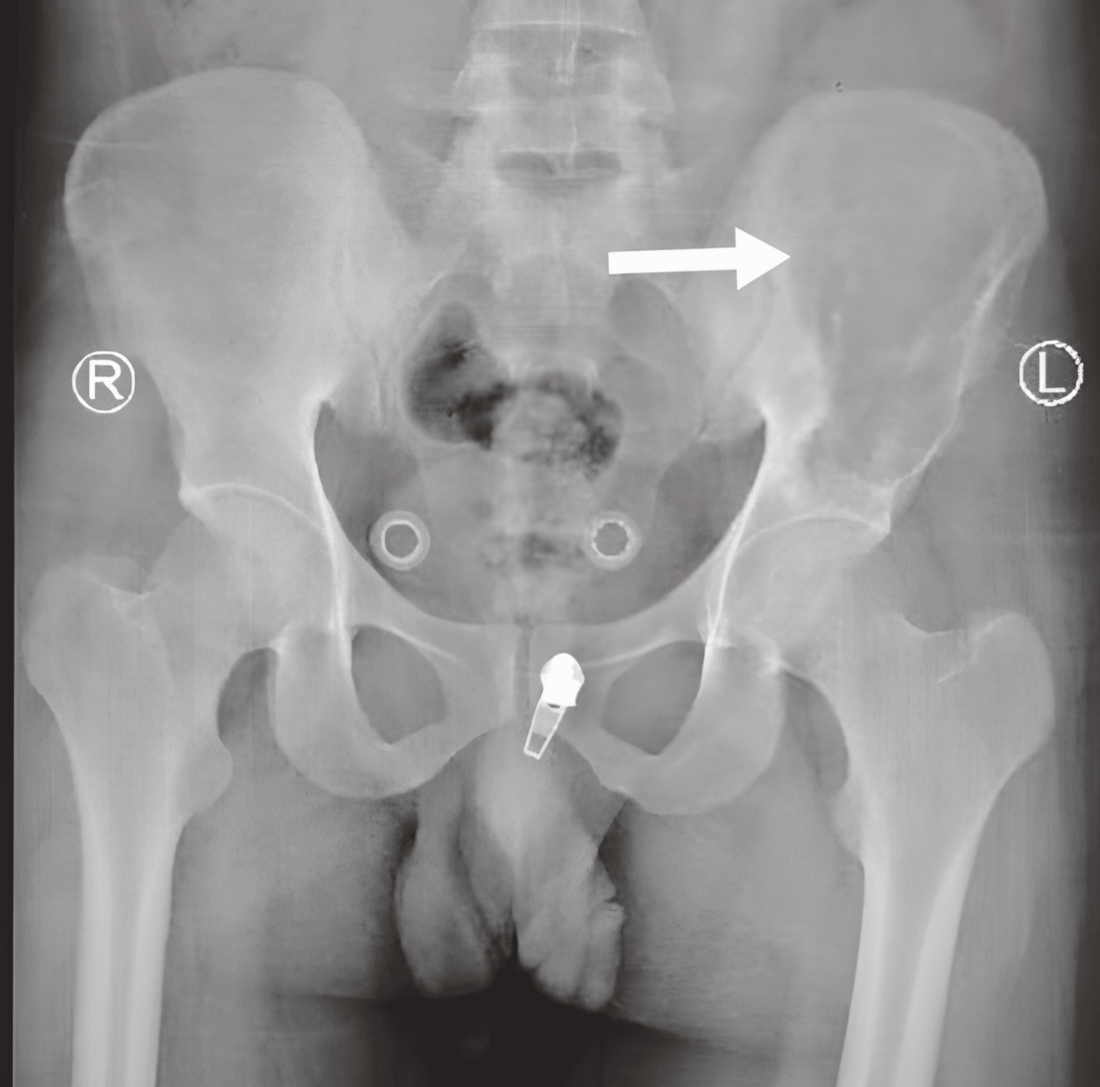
Plain X-ray of pelvis demonstrate left iliac bone lytic lesion (arrow)
